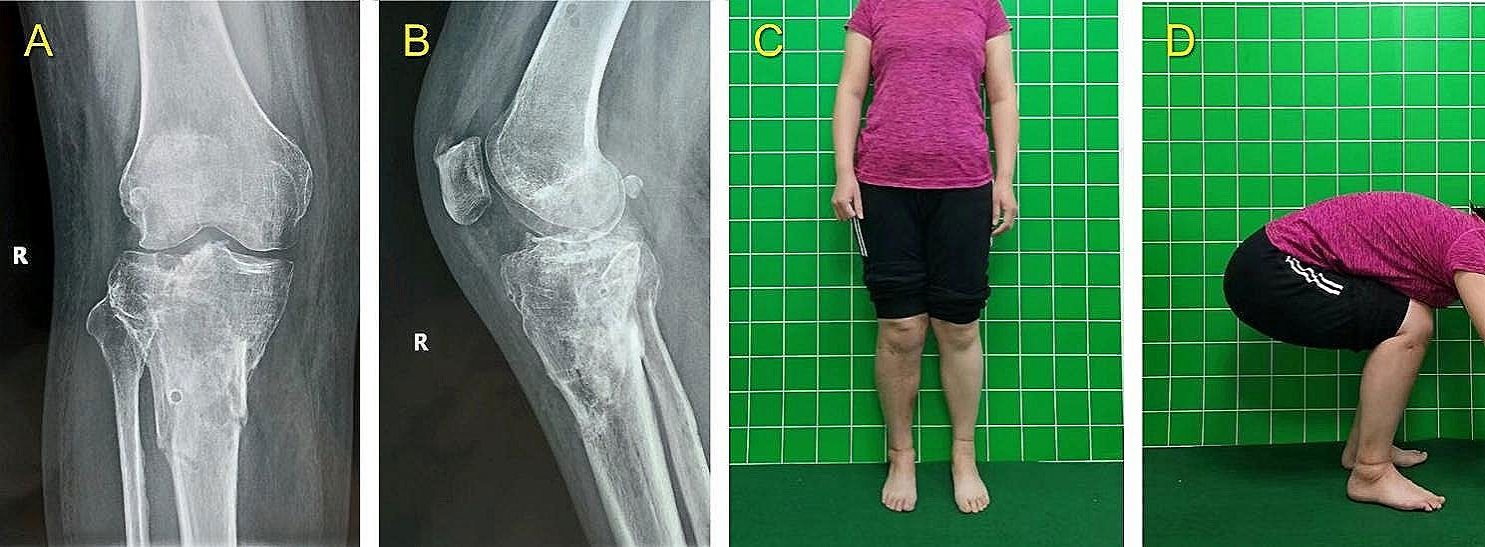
The follow-up images of the same patient shown in the Fig. 5 after removing the TSF. (A, B) AP and lateral views of X-rays 4 weeks after TSF removal. (C, D) Clinical AP and lateral views 4 weeks after TSF removal
